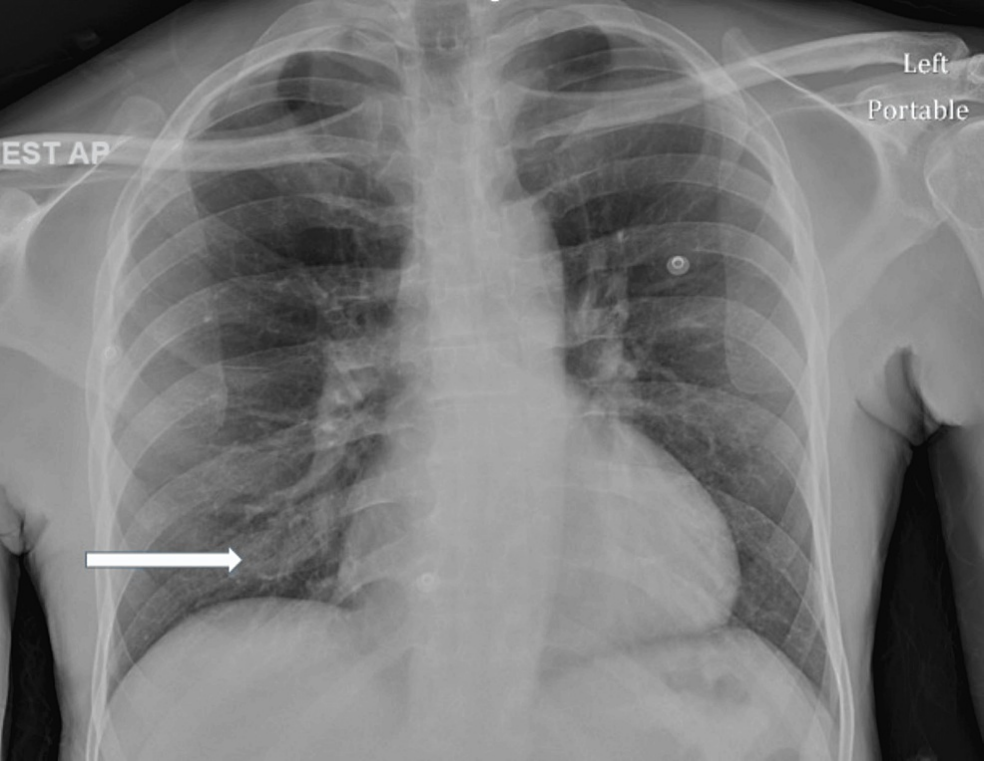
Chest X-ray showing right lower lobe infiltrates (arrow)
